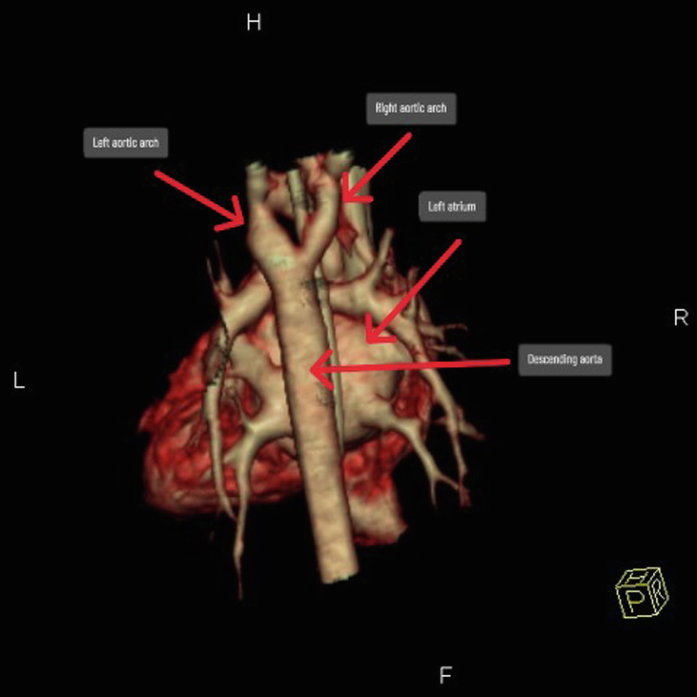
3-Dimensional Computed Tomography Angiography Reconstruction Showing a Double Aortic Arch Forming a Complete Vascular Ring
